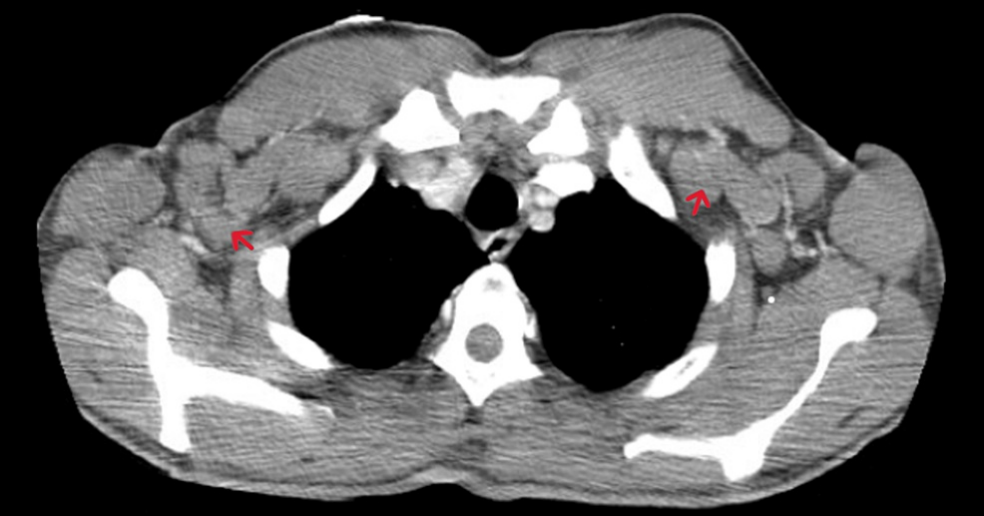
CT angiography (CTA) chest with contrast (axial) showing axillary lymphadenopathy (red arrows).
